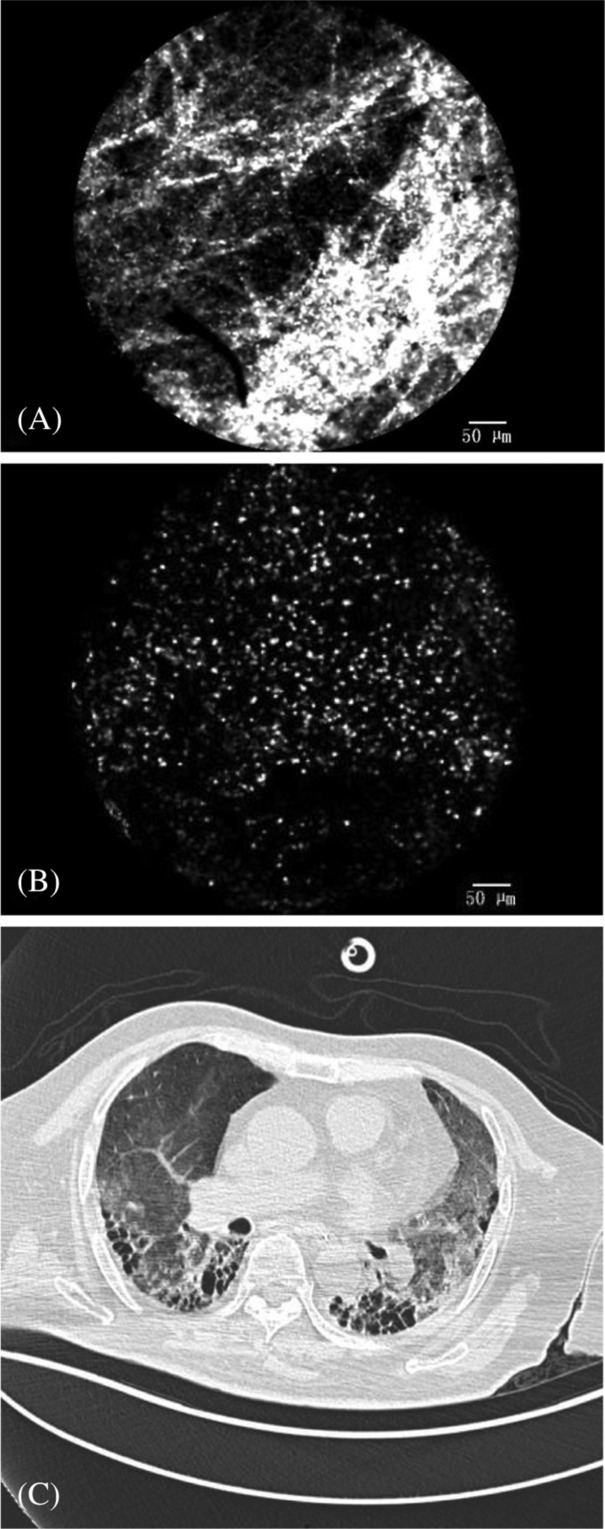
The pCLE (A, densely packed fibres and absent alveolar structures; B, numerous inflammatory cells) and CT images of one suspected dermatomyositis associated diffuse alveolar damage (DAD) patient.
